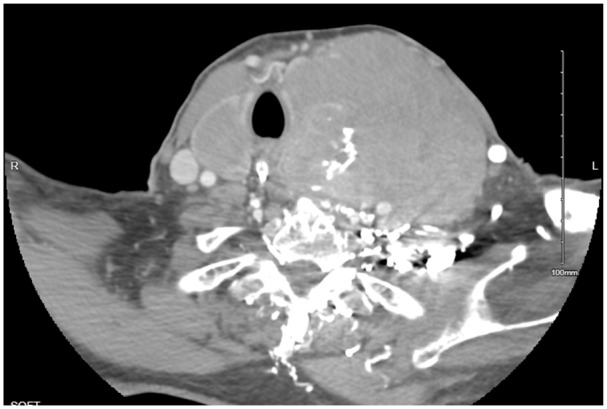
CT of the neck soft tissue with contrast: a large solid-appearing left neck mass with internal calcifications that appears similar in size up to 9.0 × 9.2 × 11 cm in size; this lesion appears to be centered within the left thyroid gland and extends into the superior mediastinum. There is a cystic nodule seen within the right thyroid lobe measuring 3.3 × 2.5 × 4.5 cm.
